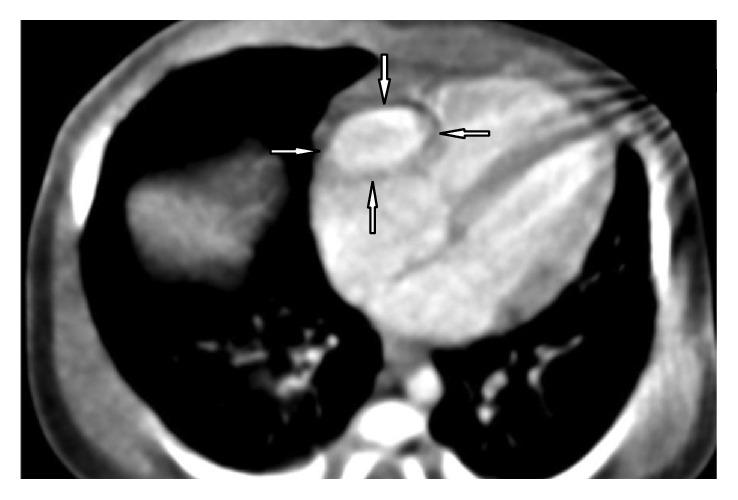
A large aneurysm of the right atrial appendage, measured in cardiac CT (marked with arrows).
